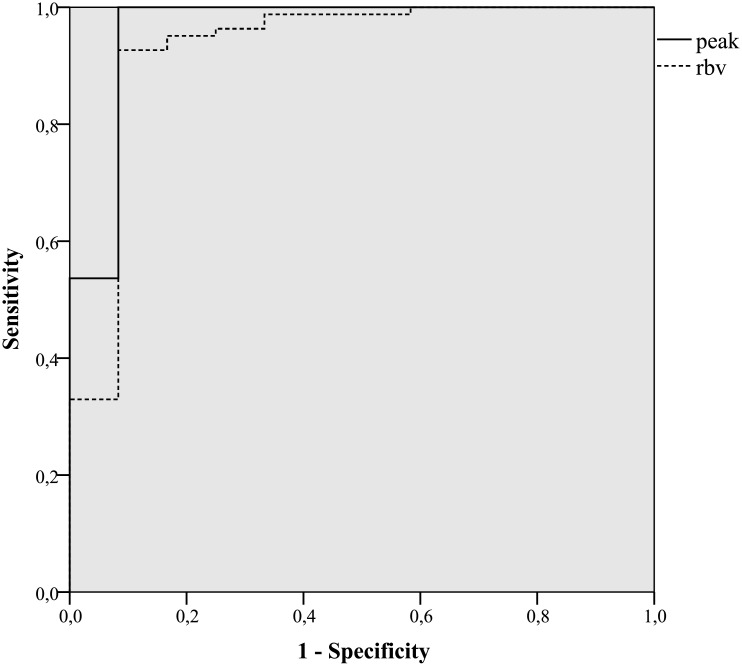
Receiver Operating Characteristic curve for performance of Contrast enhanced Ultrasound for the diagnosis of active ileitis at endoscopy. Peak intensity (solid line) and Regional Blood Volume (dotted line).
